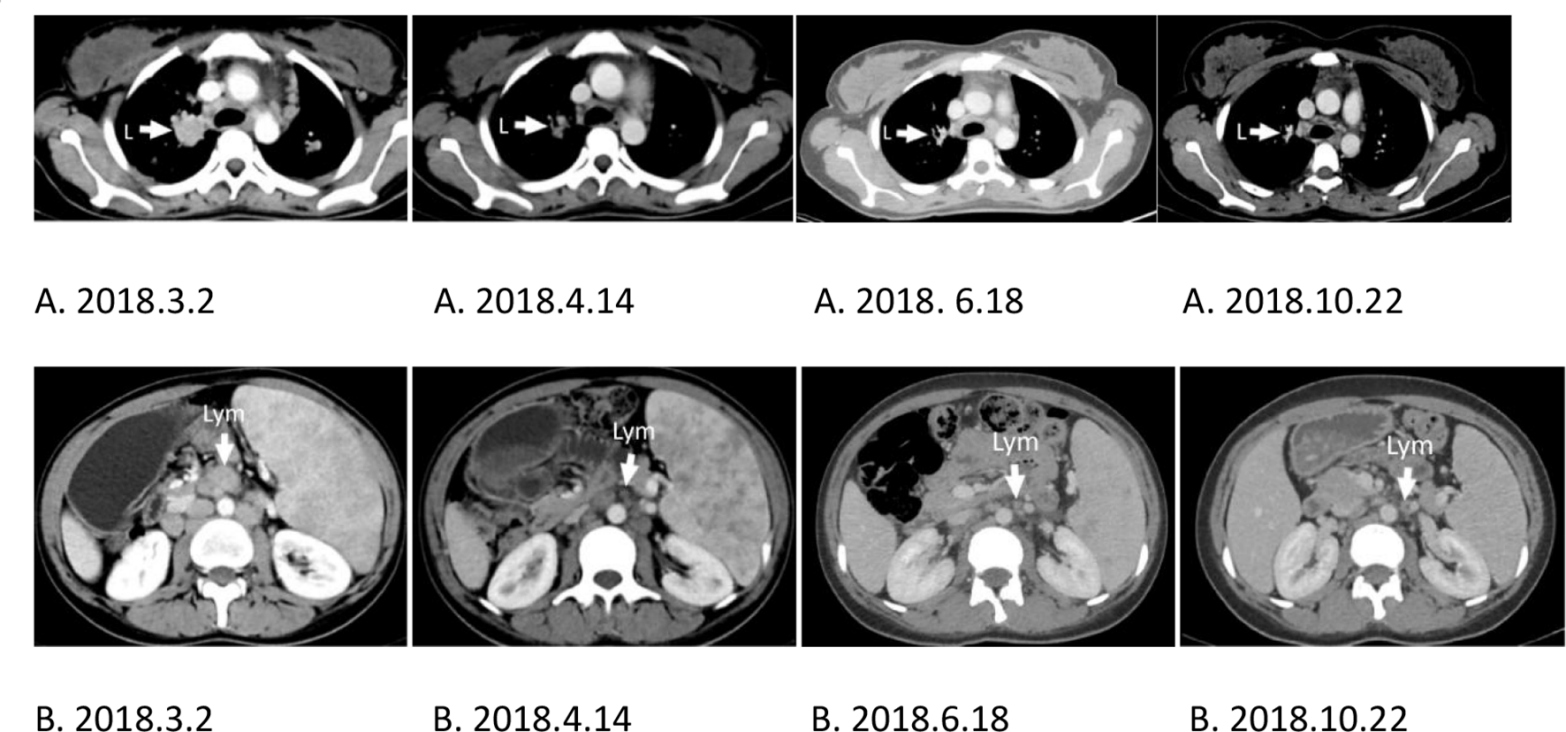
L, Lung; Lym, lymphonodus. (A, B) CT scans comparing the same location as indicated by the arrow at different times showing tumor reduction. (A) Lung metastasis. (B) Lymphadenopathy of the abdomen. (A, B) 2018.3.2. The baseline of lung metastasis or lymphadenopathy of the abdomen before CD19 CAR T-cell treatment. (A, B) 2018.6.18. The baseline of lung metastasis or lymphadenopathy of the abdomen before CD30 CAR T-cell treatment.
